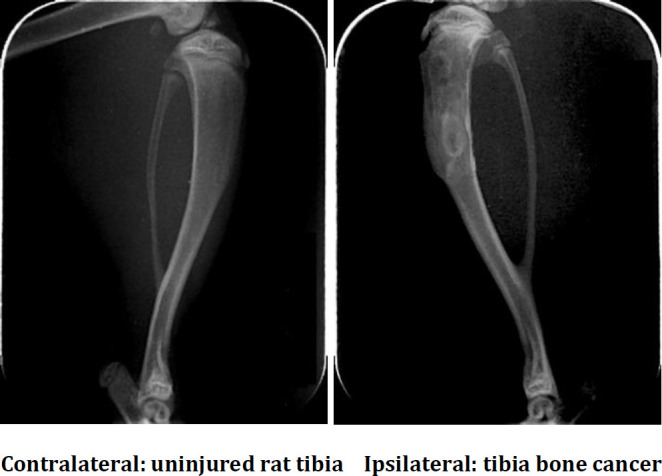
Tibia destruction after cancer cell injection. Radiography of the contralateral tibia shows an intact tibia, whereas the ipsilateral tibia shows irregular medullary cavity opacity and bone structure discontinuity proximal to the site of cancer cell injection
